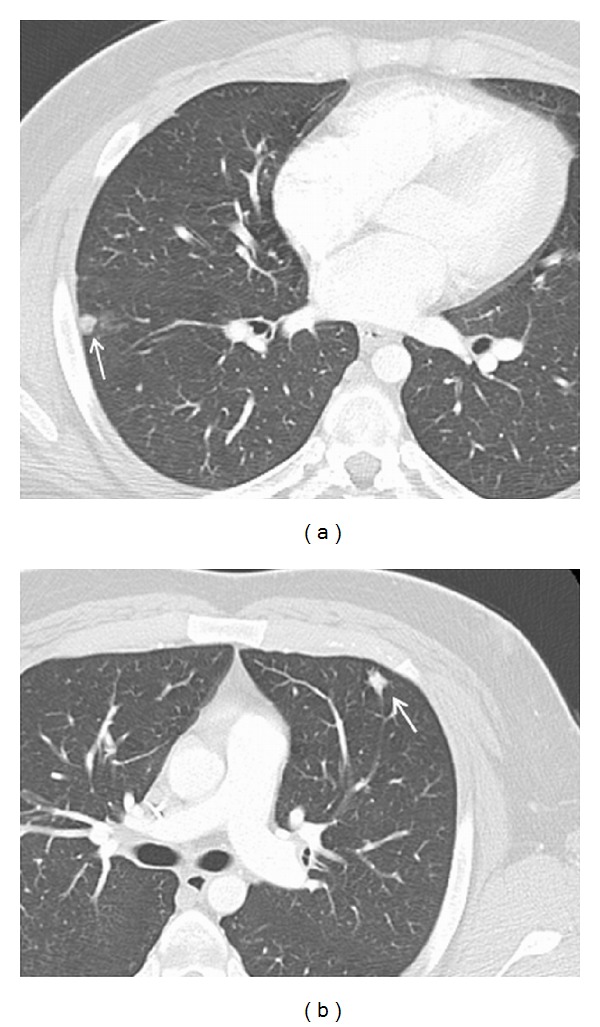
((a) and (b)) Axial CT scan images of the chest reveal small subcentimeter nodules in the right lower lobe (arrow in (a)) and left upper lobe nodule (arrow in (b)), suspicious for pulmonary metastases.
